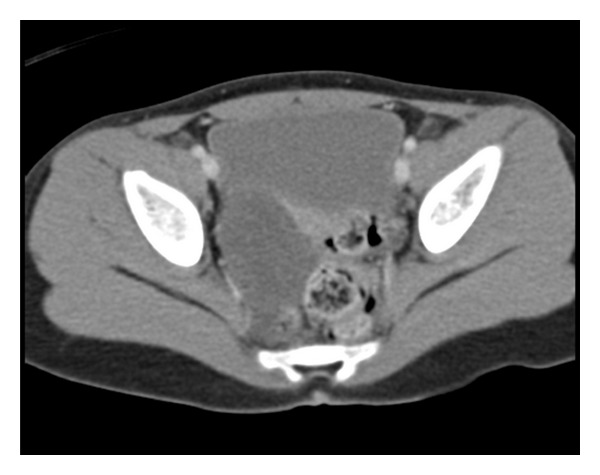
Computed tomography of the pelvis with IV contrast demonstrates a complex loculated fluid collection within right adnexa.
